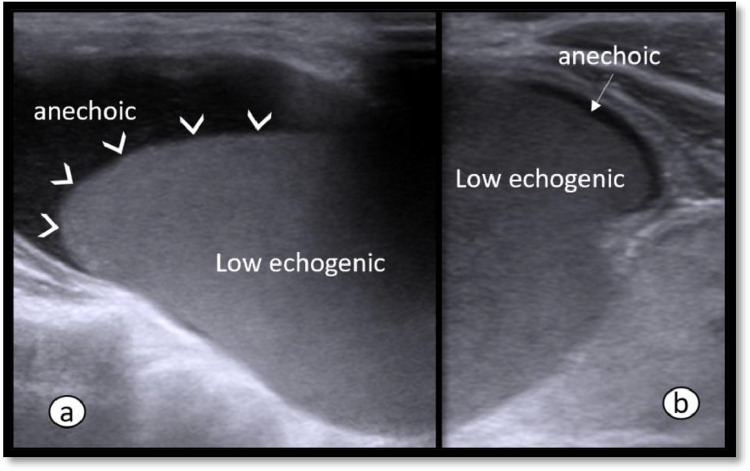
(a,b): Ultrasound showing internal content of the colloid cyst. Basal homogenous low echogenic and upper anechoic content(a,b). Arrow heads are showing curved upper margin of the basal content (a).
